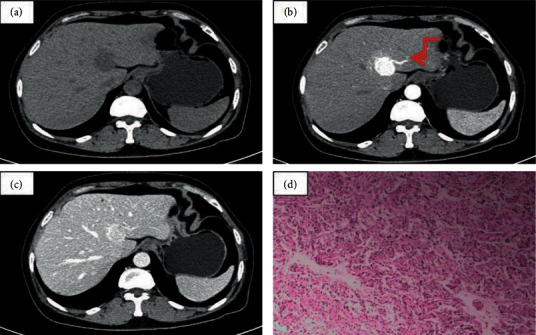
One case of a pathologically confirmed liver paraganglioma in a 60-year-old female. (a) Noncontrast CT scan showed a well-defined lesion in the liver segment IV with a mean diameter of 22 mm. (b, c) Contrast-enhanced CT scans showed homogenous and significant enhancement with the “halo sign,” peritumor feeding, or draining enlarged vessels (arrow). The CT values of the noncontrast, arterial, and venous phases were 35, 199, and 120 HU, respectively. The lesion was misdiagnosed as benign hemangioma or FNH tumour following preoperative CT. (d) The tumor cells were arranged in nests, surrounded by fibrovascular stroma.
